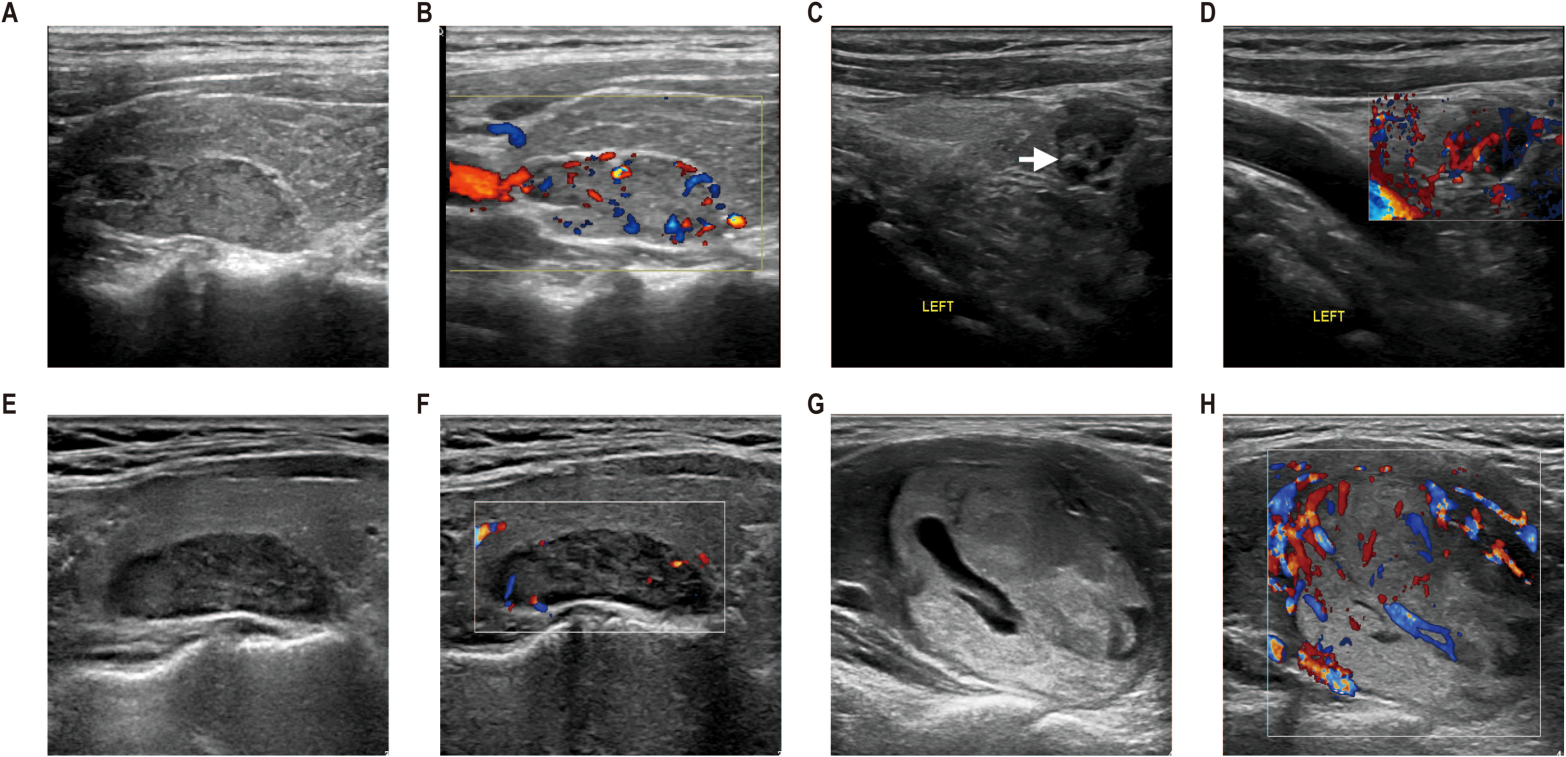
The ultrasonographic manifestations of parathyroid lesions with different pathological types. (A, B) Ultrasonography from a 27-year-old man with parathyroid carcinoma (PC). An isoechoic solid lesion with an incomplete capsule and irregular shape was located in the right upper parathyroid region, with the relation to the thyroid gland extending >50%. Color Doppler flow imaging (CDFI) revealed polar vessels with increased mixed vascularity. (C, D) Ultrasonography of a 54-year-old woman with APT. A hypoechoic solid lesion with incomplete capsule, irregular shape, and internal calcification (arrows) was observed in the lower left parathyroid region, with the relation to the thyroid gland extending ≤50%. CDFI demonstrated polar vessels with increased mixed vascularity. (E, F) Ultrasonography of a 51-year-old woman with parathyroid adenoma. Ultrasound examination revealed a hypoechoic lesion with complete capsule and regular shape in the upper left parathyroid region. The relation to the thyroid gland extended >50%. CDFI, avascularity. (G, H) Ultrasonography of a 49-year-old woman with parathyroid adenoma. A mixed echotexture lesion with cystic change <50% was identified in the lower left parathyroid region, exhibiting a complete capsule and regular shape. The relation to the thyroid gland extended >50%. Postoperative pathology confirmed parathyroid adenoma.
